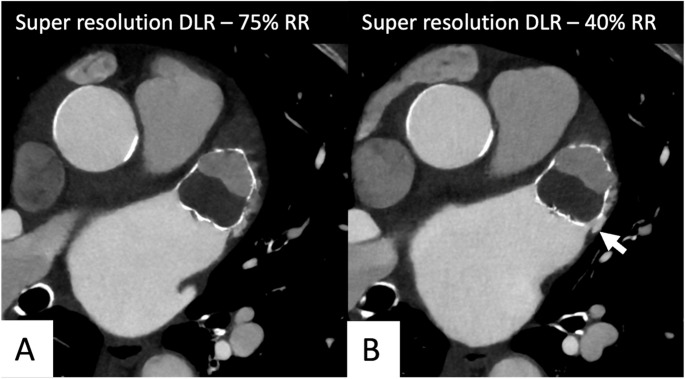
Cardiac CT images demonstrating dynamic peri-device leak following Watchman device implantation. (A) Diastolic phase (75% R-R interval) images show the device in appropriate position with presence of contrast in the excluded portion of the left atrial appendage; however, PDL location and size could not be determined. In systolic phase (40% R-R interval, B) PDL location (arrows) becomes apparent due to left atrial wall motion
